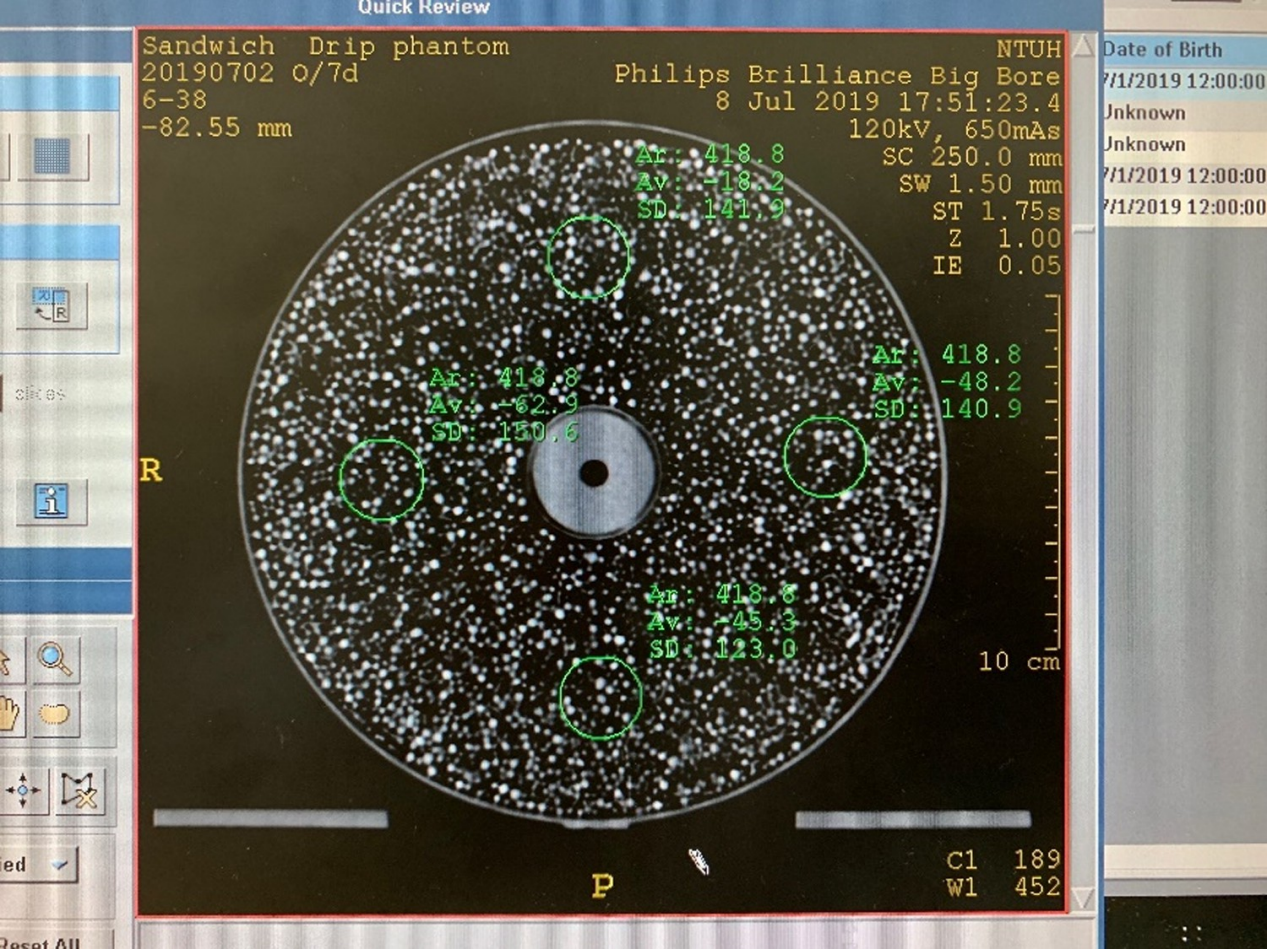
CT image of the silicone-filled uniformity slab.
